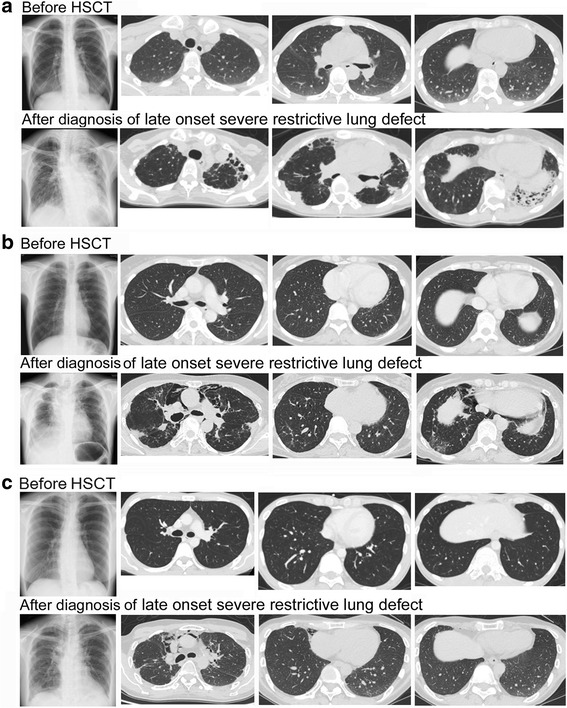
Representative CT findings of PPFE pattern in patients with late-onset severe restrictive lung defect. Chest radiograph and chest CT of representative cases [a) case No. 2, b) No. 4, and c) No. 12] with late-onset severe restrictive lung defect cases before HSCT and after diagnosis of late-onset severe restrictive lung defect are shown
